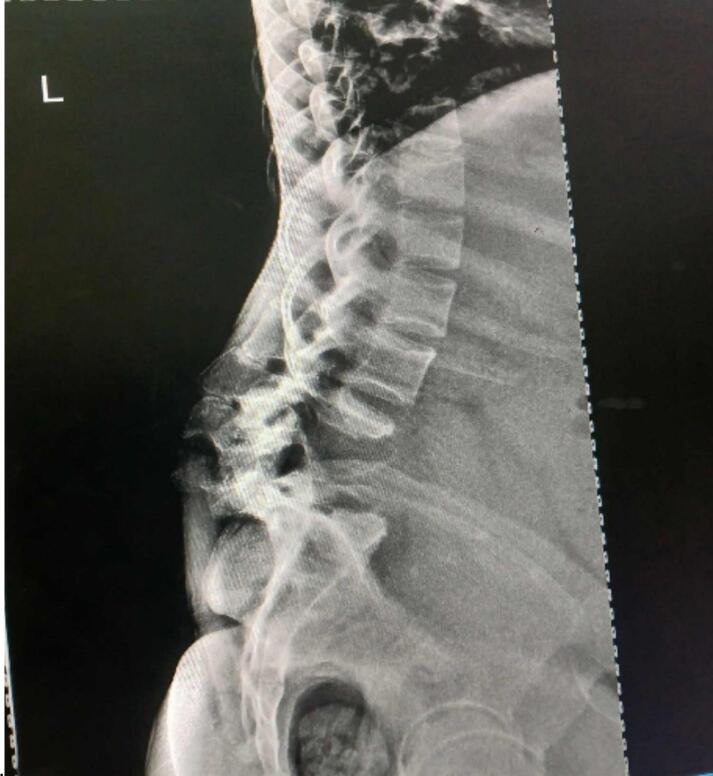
Lumbar spine X-ray demonstrated severe kyphotic deformity and destruction of the lower lumbar vertebrae (L4-L5), with wedging and sclerosis of L3 and a perivertebral soft tissue mass.
